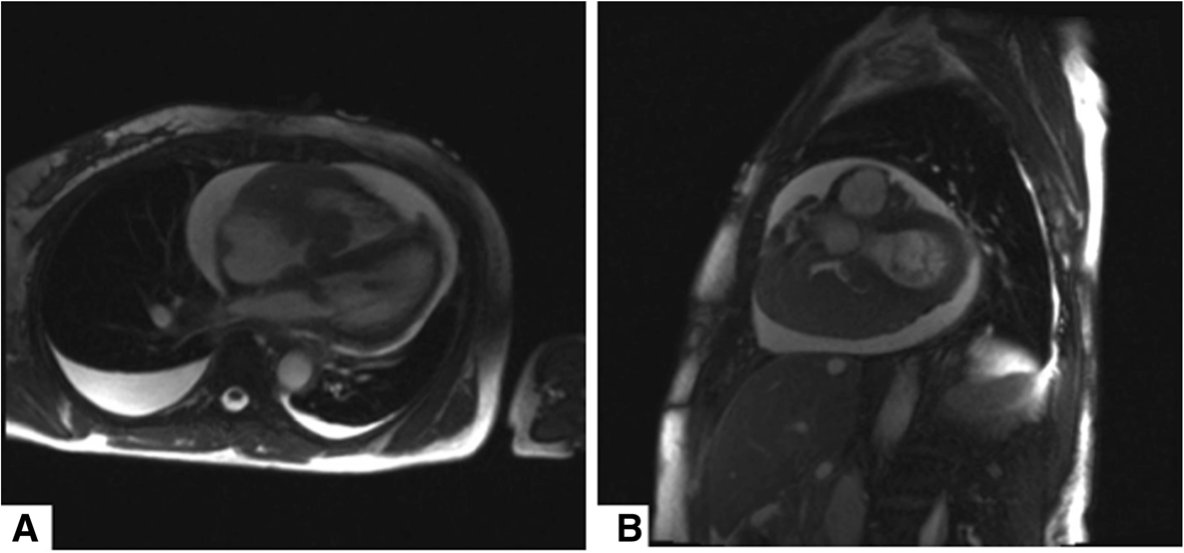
Magnetic resonance imaging demonstrating no evidence of mediastinal lymphadenopathy. A Axial view, T2 weighted images showing both pericardial and bilateral pleural effusions, right greater than left. Right ventricular wall irregularities and right greater than left intracavitary tumor formation, partially obstructing the right atrial and the right ventricular flow. B Sagittal view, T2 weighted images evidencing intracavitary tumor formation and both pericardial and bilateral pleural effusions. No evidence of mediastinal lymphadenopathy could be observed.
